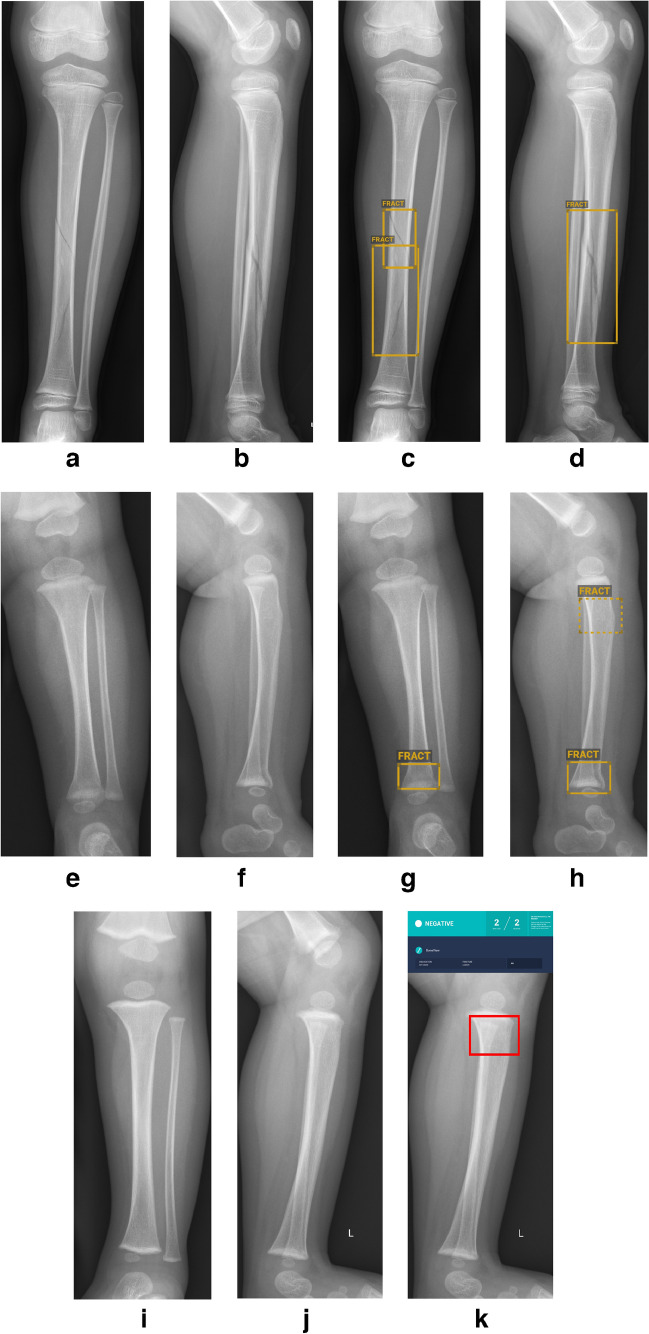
Examples of lower leg radiographs showing true positive (a–d), false positive (e–h) and false negative (i–k) diagnoses made by BoneView (Gleamer, Paris, France). a–d Anteroposterior (AP) and lateral radiographs of the left lower leg in a 5-year-old girl before (a,b) and after (c,d) annotation by BoneView. There is a tibial spiral fracture. The fracture was detected with a confidence score above 90% (*rectangles/“FRACT”*). e–h AP and lateral radiographs of the left lower leg in an 11-month-old girl before (e,f) and after (g,h) annotation by BoneView. There is a distal tibial Salter-Harris-II fracture. The true positive finding of a fracture was detected with a confidence score above 90% (*rectangles/“FRACT*”). Additionally, BoneView detected a false positive proximal fracture on the lateral radiograph (h) with a confidence score between 50% and 90% (*broken rectangle/“FRACT”*). i–k AP and lateral radiographs of the left lower leg in a 1-year-old boy before (i,j) and after (k) annotation by BoneView. There is a proximal metaphyseal tibial buckle fracture (trampoline fracture), which is only visible on the lateral radiograph (*rectangle*). BoneView did not detect the fracture
